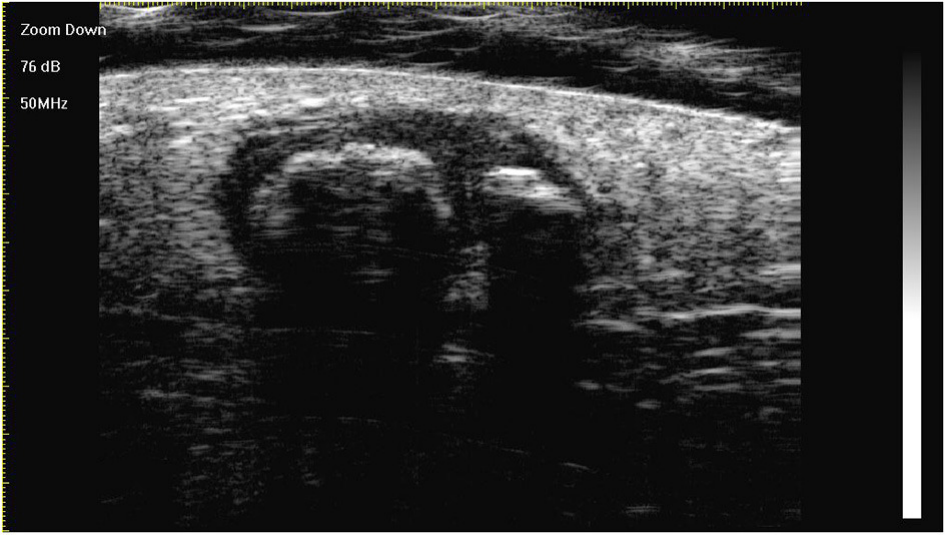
The lesion is a heterogeneous, well-demarcated, hypoechoic tumour located between the deep dermis and the subcutis with typical ultra-high-frequency ultrasonographic features, including internal echogenic foci, hypoechoic rim, and posterior acoustic shadowing.
